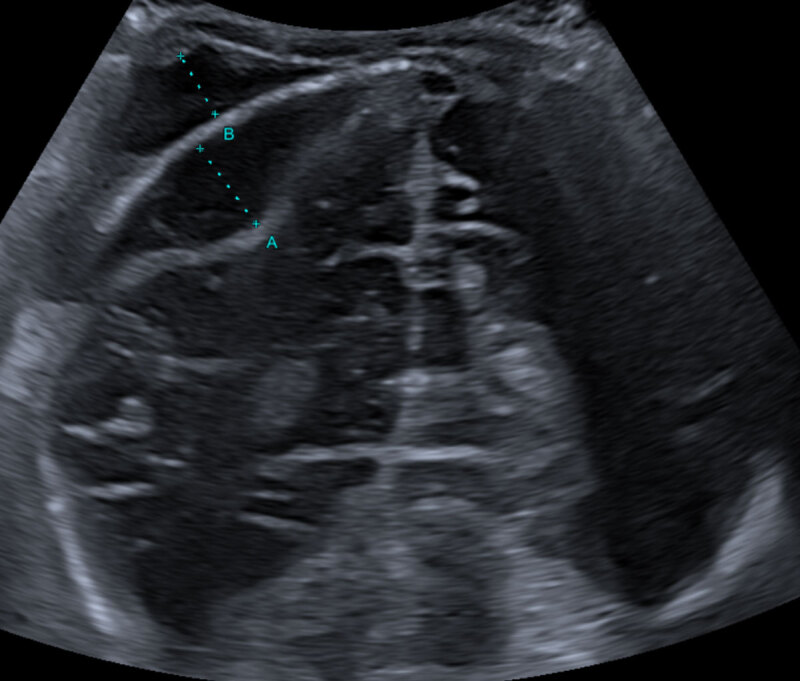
Because angulation of the probe could not clearly eliminate the possible presence of an epidural hematoma, in consultation with the treating paediatrician it was opted to further evaluate the infant through non-contrast-enhanced computed tomography (NECT) of the brain. Coronal NECT image (*Figure 2*) at the same level as the ultrasound image in *Figure 1* shows the cephalhematoma without underlying intracranial abnormalities.
